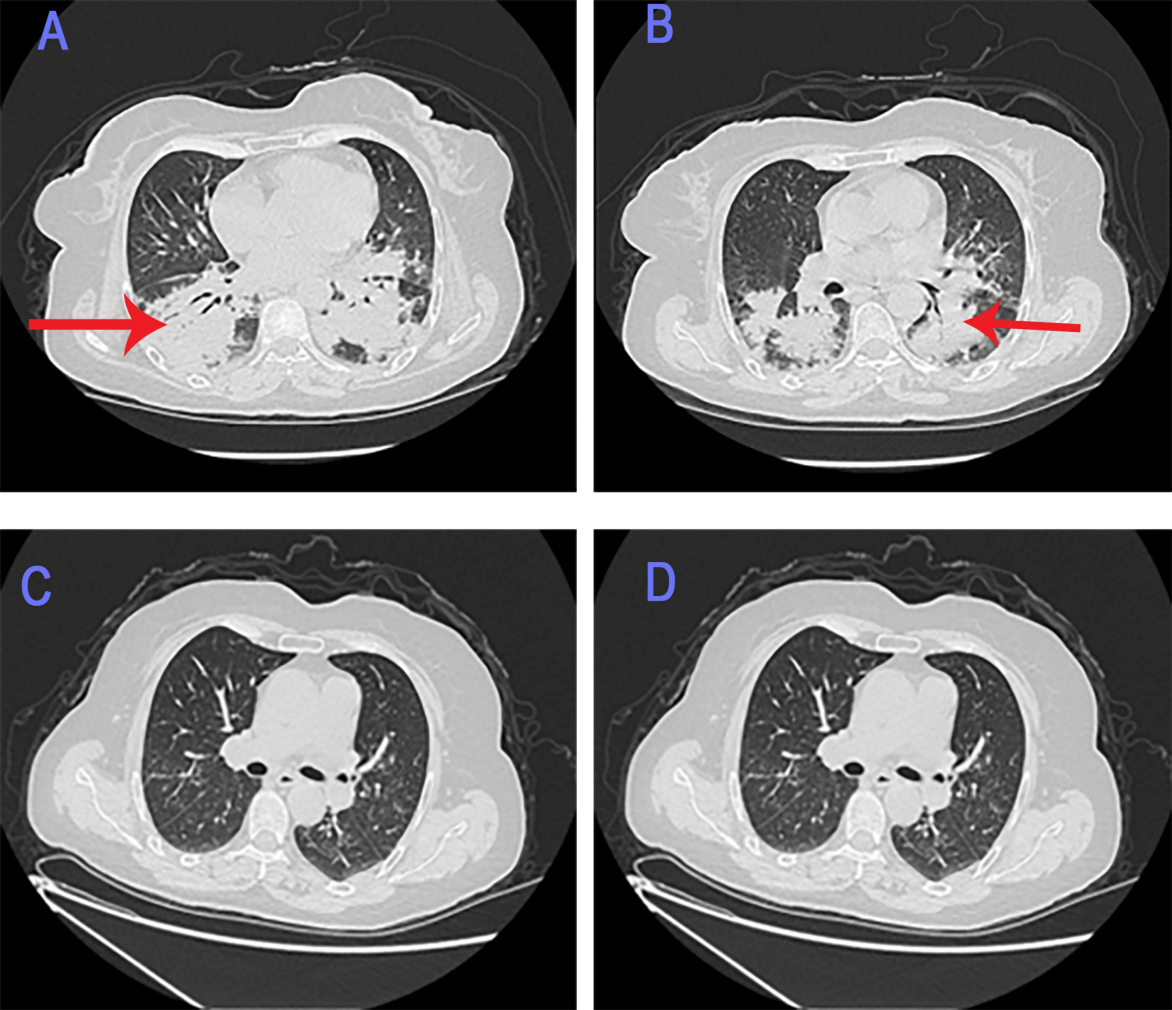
Chest CT scan. Chest CT scan showing bilateral alveolar syndrome (red arrow) before treatment (A and B) and resolution of the alveolar syndrome after treatment (C and D).
